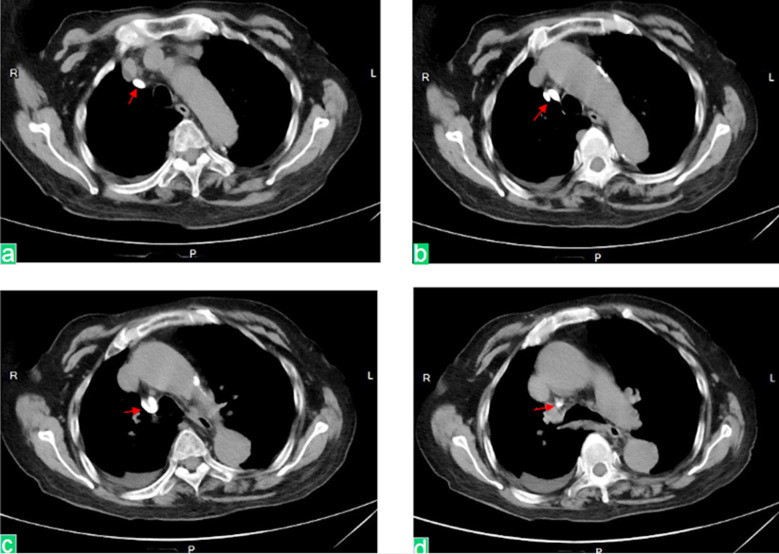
Scan of the chest CT from top to bottom shows the dialysis catheter penetrating the superior vena cava and into the mediastinum. Red arrows indicate the dialysis catheter.
